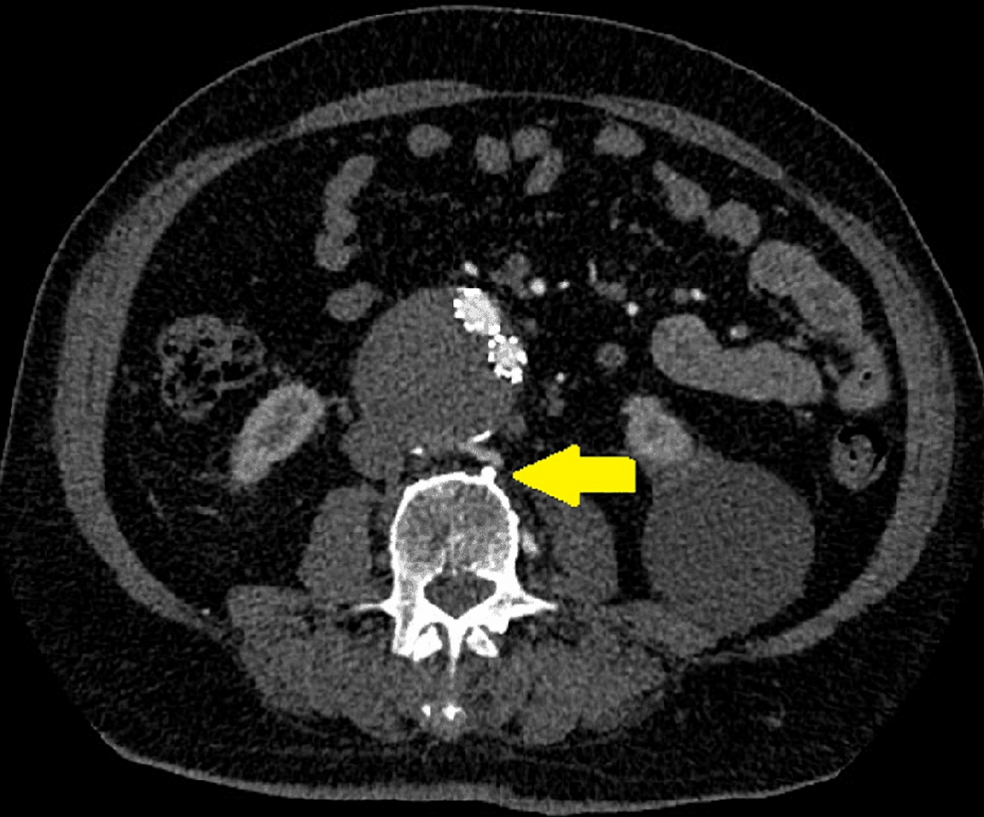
Pre-operative computed tomography angiography shows patent lumbar artery leading to endoleak type II.
